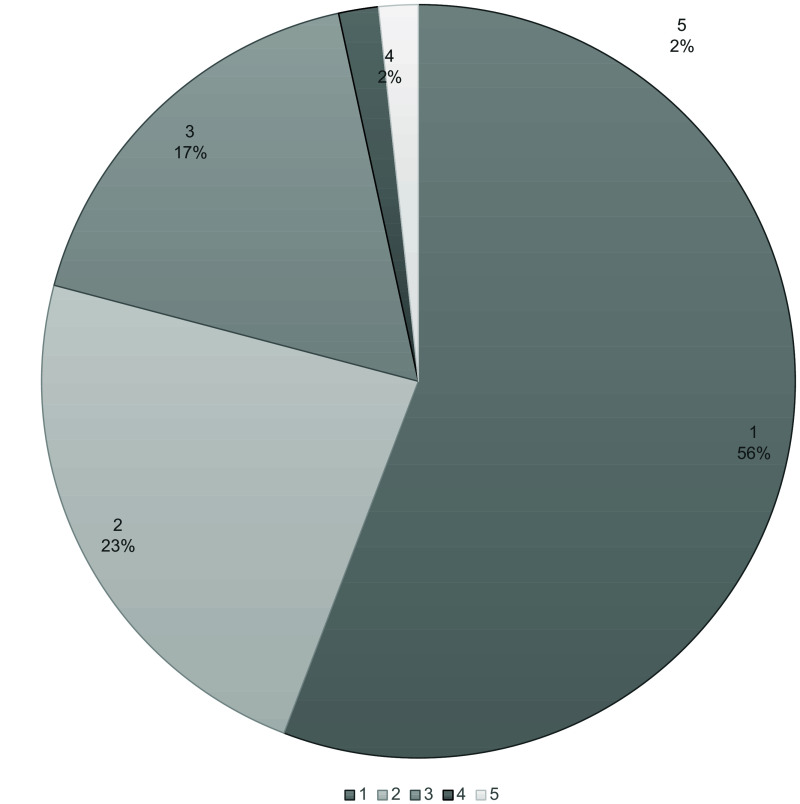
Results of the question “confident that marking in DTS corresponds to the marking CT.” 1 = Confident that the statement is true. 2 = Somewhat confident that the statement is true. 3 = I do not know if the statement is true or not. 4 = Somewhat confident that the statement is not true. 5 = Confident that the statement is not true. Abbreviations; DTS = digital tomosynthesis and CT = computed tomography.
